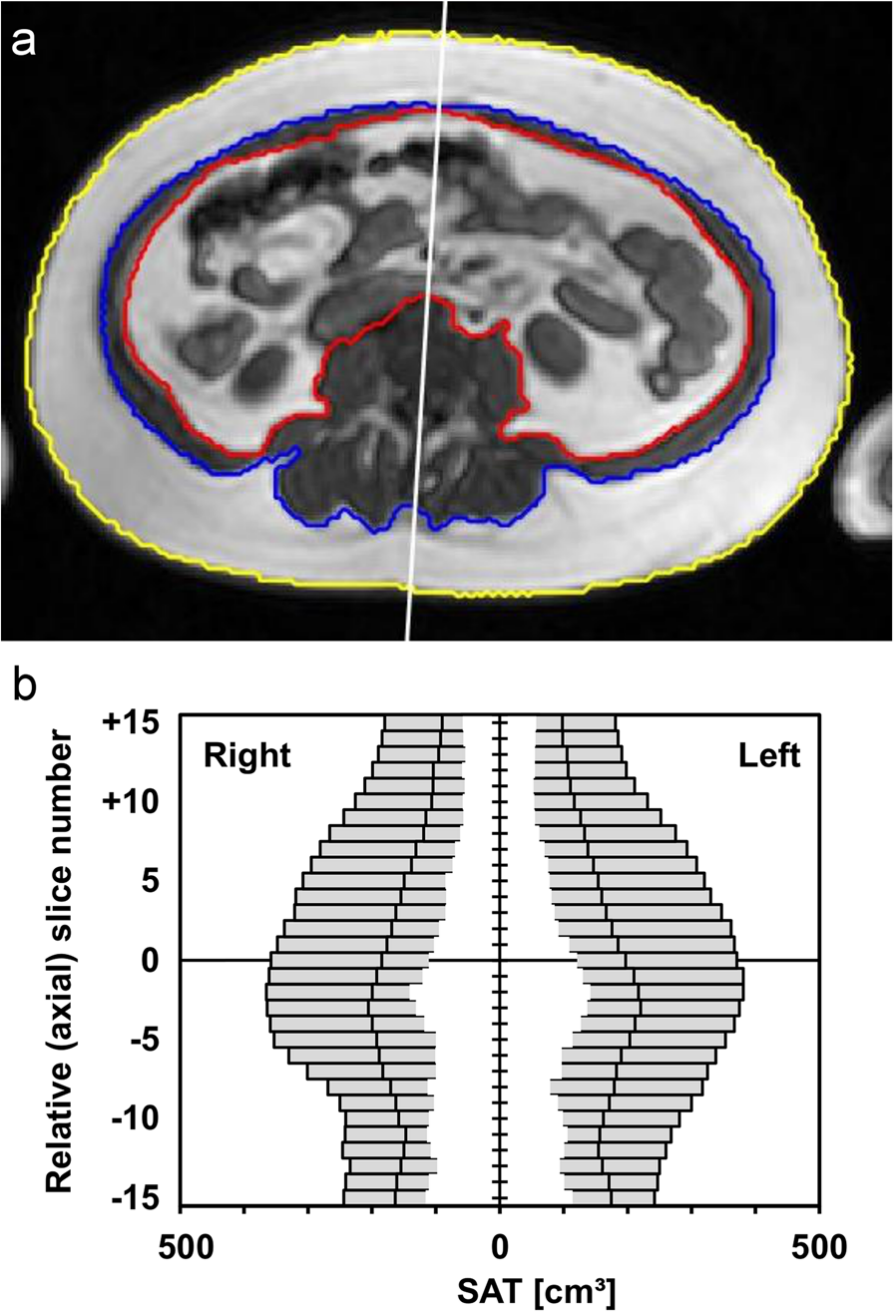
Quantification of abdominal adipose tissue in MRI. a Screenshot of the segmentation software (Matlab). The manually drawn median line is meant to separate the two body halves. Colored lines mark the outer (yellow) and inner (blue) ASAT boundaries and a contour (red) encompassing the VAT components. The tool is available from an online repository (https://github.com/Stangeroll/Dicomflex). b Distribution of partial ASAT volumes for left and right body halves as a function of relative (axial) slice number for all subjects (slice spacing: 10.5 mm). Outer, middle and inner vertical marks represent maximum, median and minimum values. Slice position 0 corresponds to the level of the umbilicus
